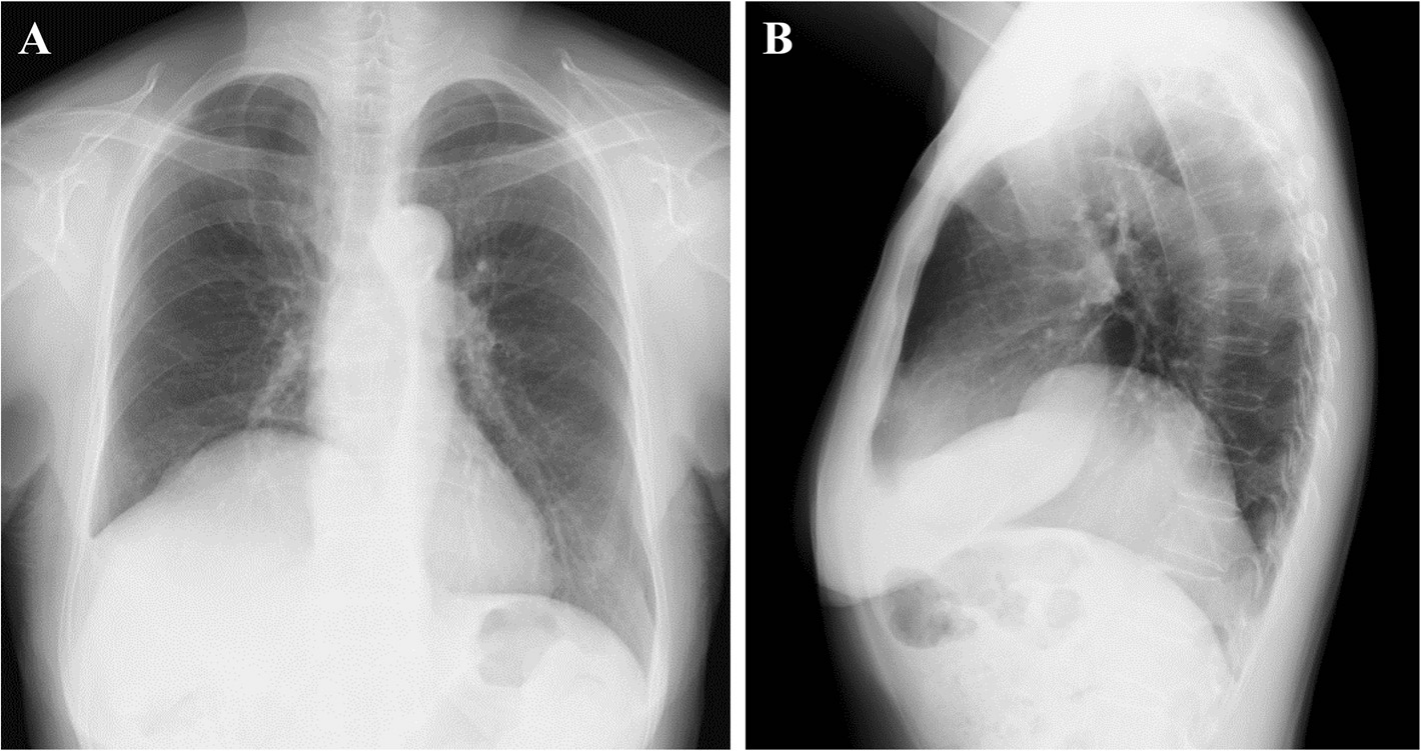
a, b Preoperative chest X-ray reveals an elevated right hemidiaphragm
